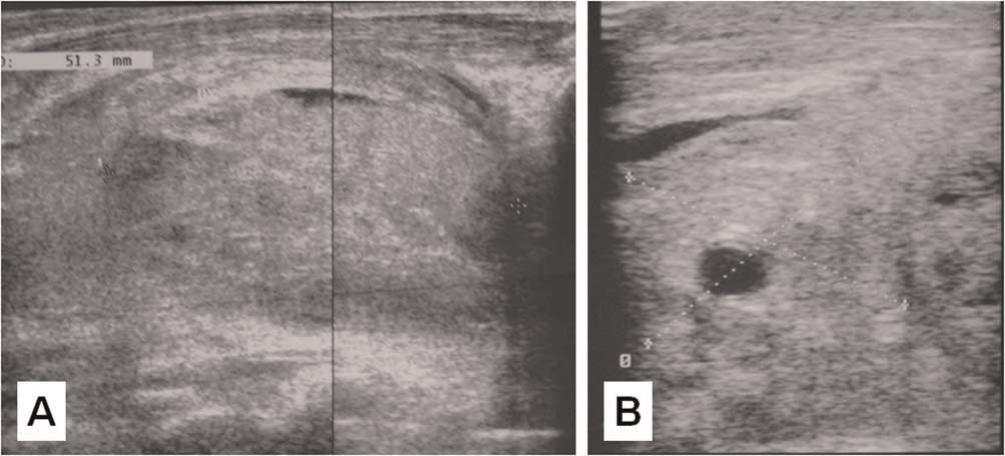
Neck ultrasound on admission. Longitudinal and transverse views demonstrating a 51.3 mm nodular iso-echoic lesion, of dyshomogeneous structure and hemorrhagic pattern (A). The lesion surrounds the right common carotid artery and internal jugular vein, and is located posterior to the right lobe of the thyroid, with ill-defined posterior margins (B).
